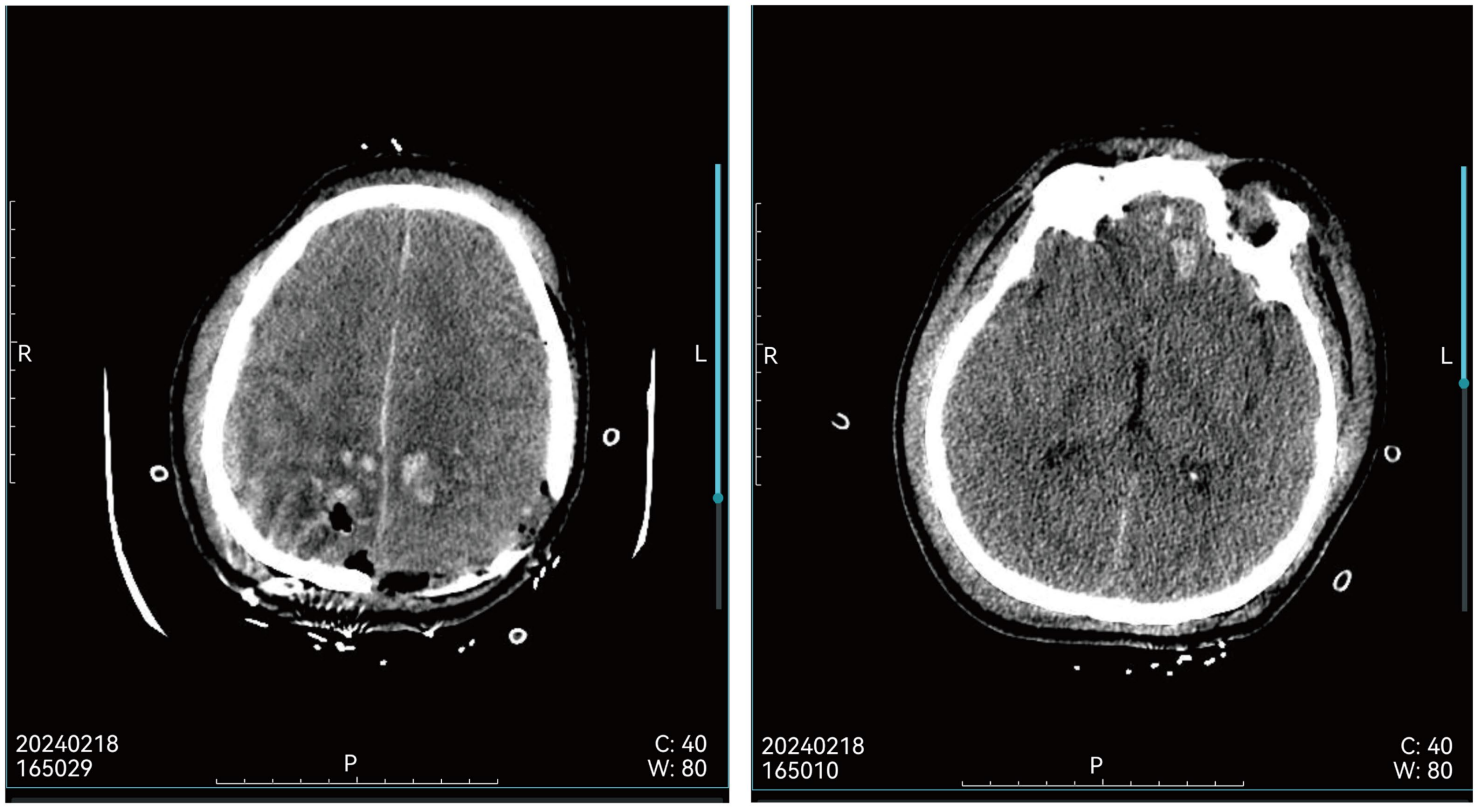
Head CT showed multiple cerebral injuries in the bilateral parietal lobes, left frontal lobe, and left temporal lobe after trauma.
